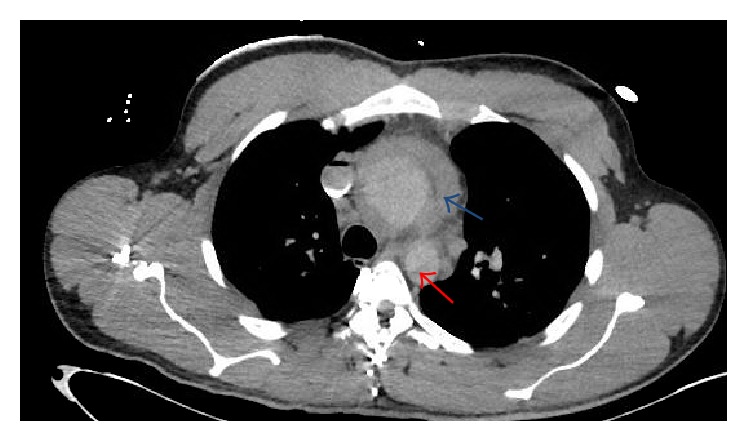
CT chest: ascending aortic dissection (red arrow) and hemopericardium (blue arrow).
